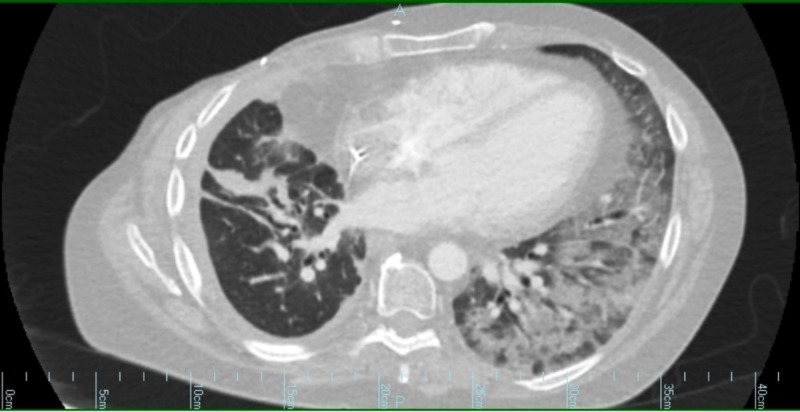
Computed tomography (CT) scan of the chest. A CT scan of the chest demonstrating consolidation and ground glass opacities predominately involving the left lung.
